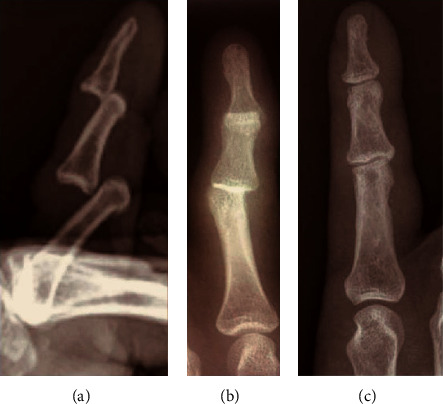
Anteroposterior and lateral radiographs (a, b) demonstrating dorsal dislocation of both proximal and distal interphalangeal joints, without any bony avulsion fracture. Postreduction radiograph (c) confirms that all joints are well reduced.
